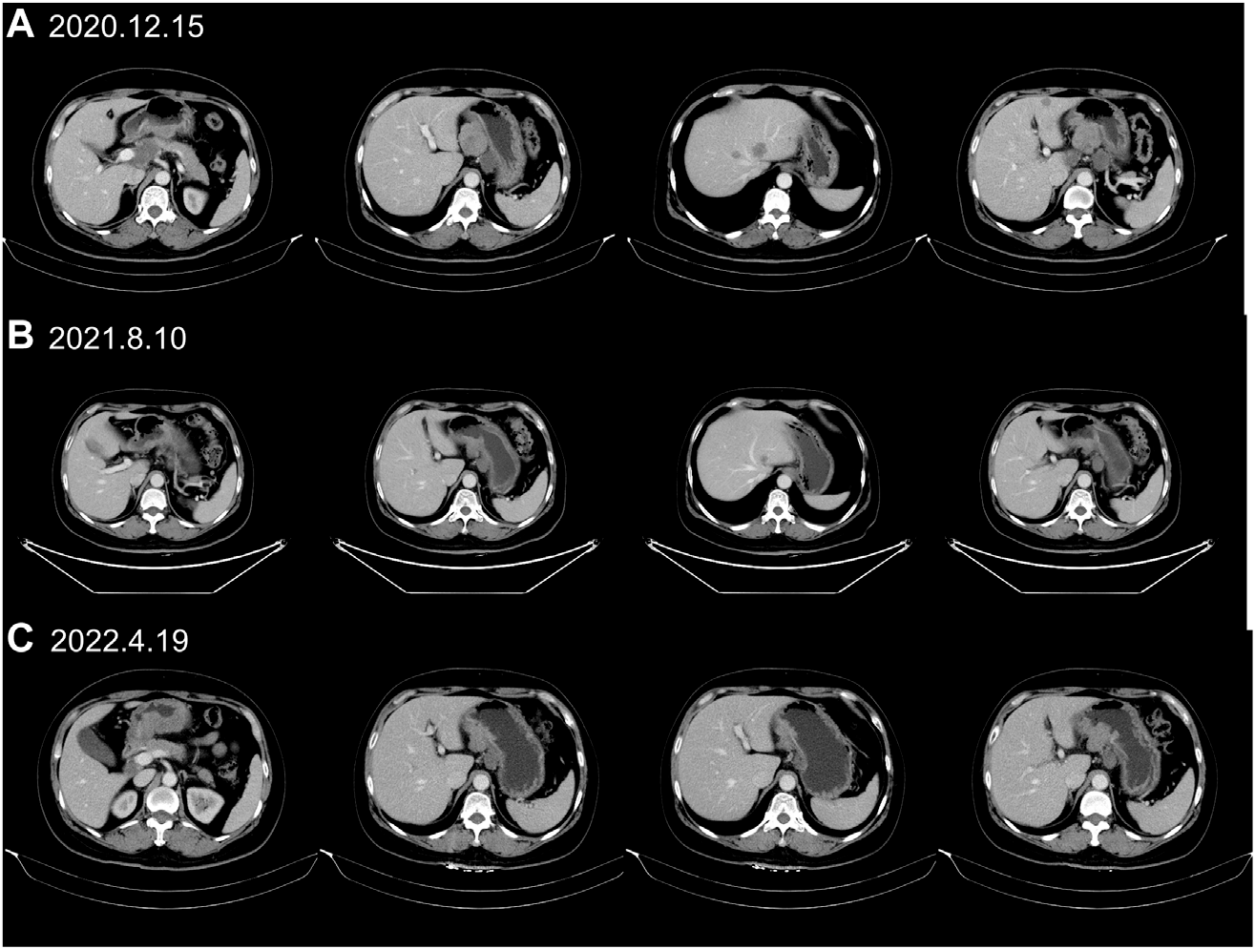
CT scans identified liver lesions during trastuzumab treatment. (A) CT scans (2020.12.15) demonstrated progressive disease (PD) in liver metastases after trastuzumab plus chemotherapy treatment. (B) CT (2021.8.10) showed that liver lesions had markedly shrunken 8 months after trastuzumab, apatinib and camrelizumab treatment. (C) The PR status (2022.4.19) continued with trastuzumab, apatinib and camrelizumab treatment until upper gastrointestinal bleeding happened.
